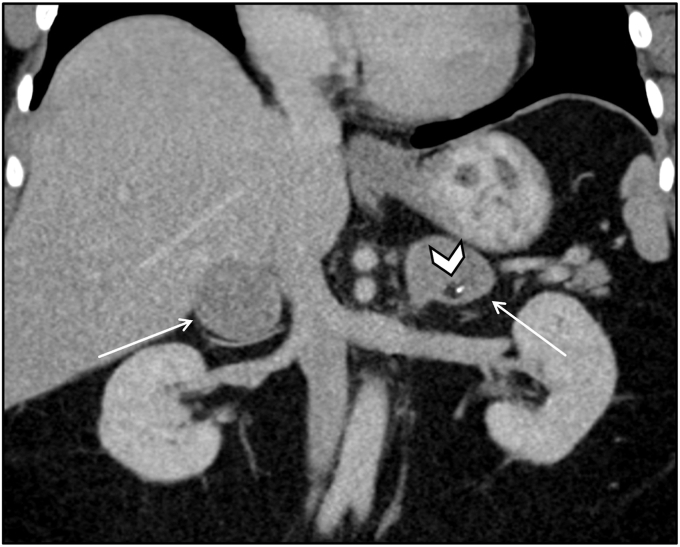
Bilateral adrenocortical neoplasms in suspected Li-Fraumeni syndrome. Coronal post contrast CT image showing bilateral adrenal lesions (arrows). The *right* adrenal lesion demonstrates heterogenous enhancement and was consistent with an adrenocortical carcinoma. The *left* adrenal lesion is heterogenous with foci of fat and calcifications (arrowhead) corresponding to sites of myelolipomatous change within this atypical adenoma. *CT* = computed tomography.
